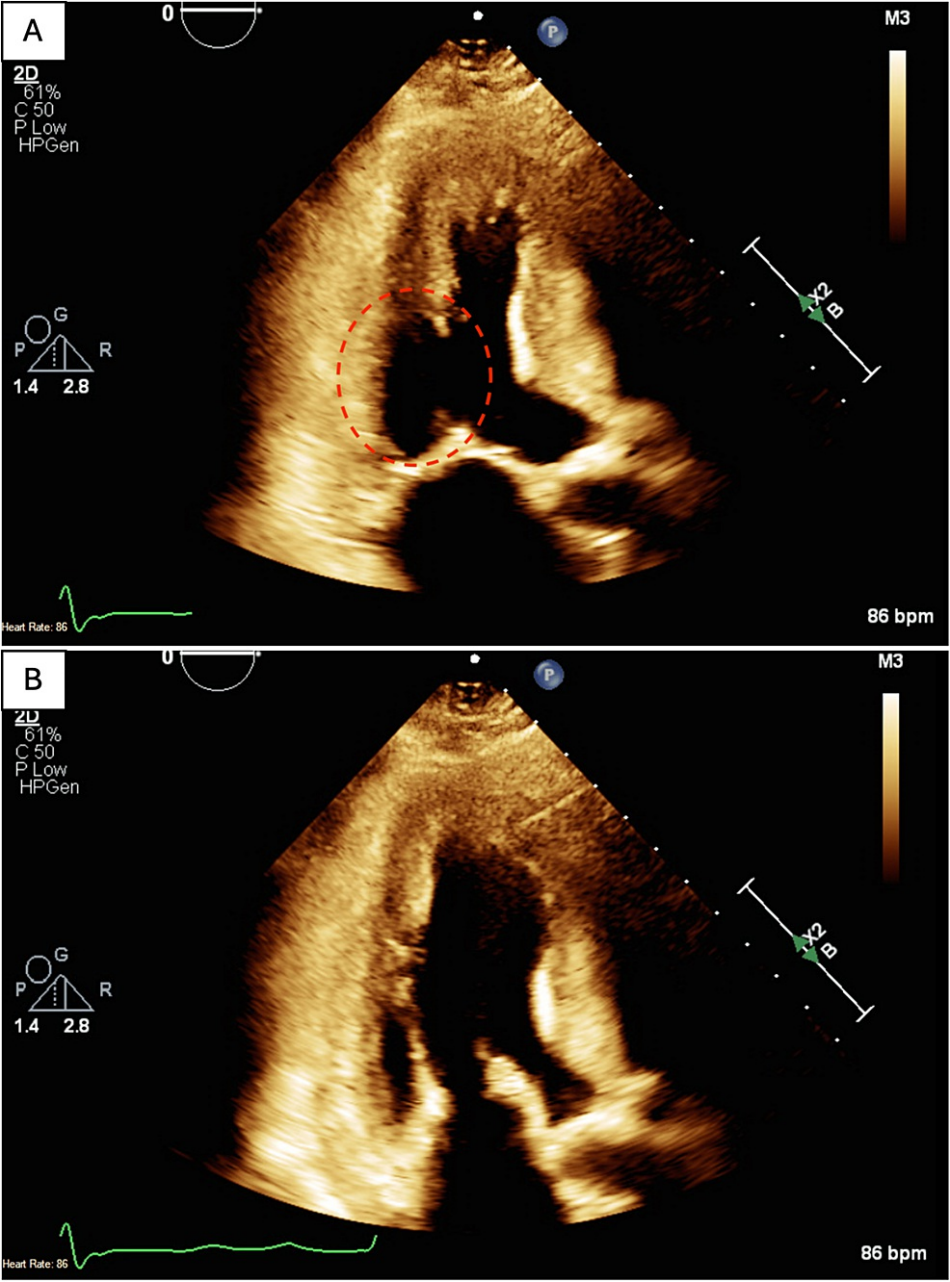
Two-chamber view echocardiogram showing the left ventricle and the left atrium. A. Displays the left ventricle during systole with akinesia in the inferolateral segment (red-dashed circle). B. Displays the left ventricle expanding during diastole.
